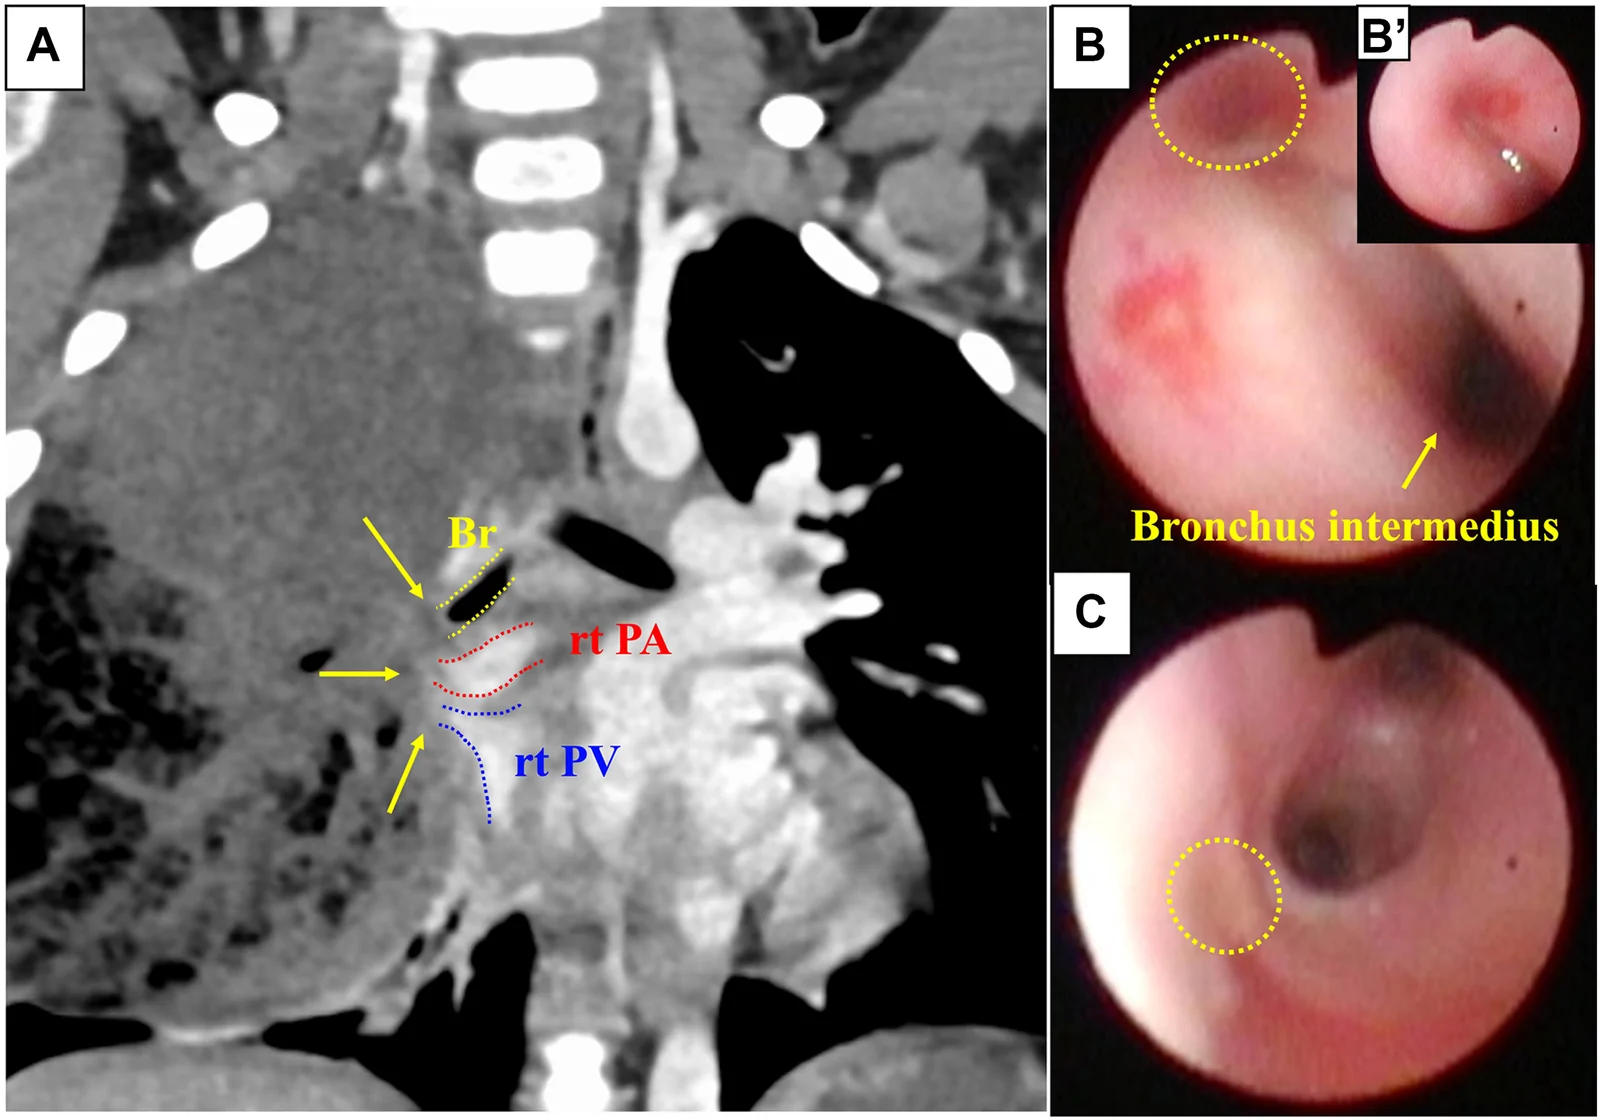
(A) Contrast-enhanced computed tomography of the chest demonstrating the “beak sign” at the right hilum involving the bronchus (Br), pulmonary artery, and pulmonary vein to the right upper and middle lobes (arrows; rt PA, right pulmonary artery; rt PV, right pulmonary vein). (B, C) Flexible bronchoscopy showing obstruction of the right upper and middle lobe bronchi indicated by circle and adherence of bloody sputum. (B′) Magnified view of the circled area in (B), showing a “fish-mouth” orifice.
